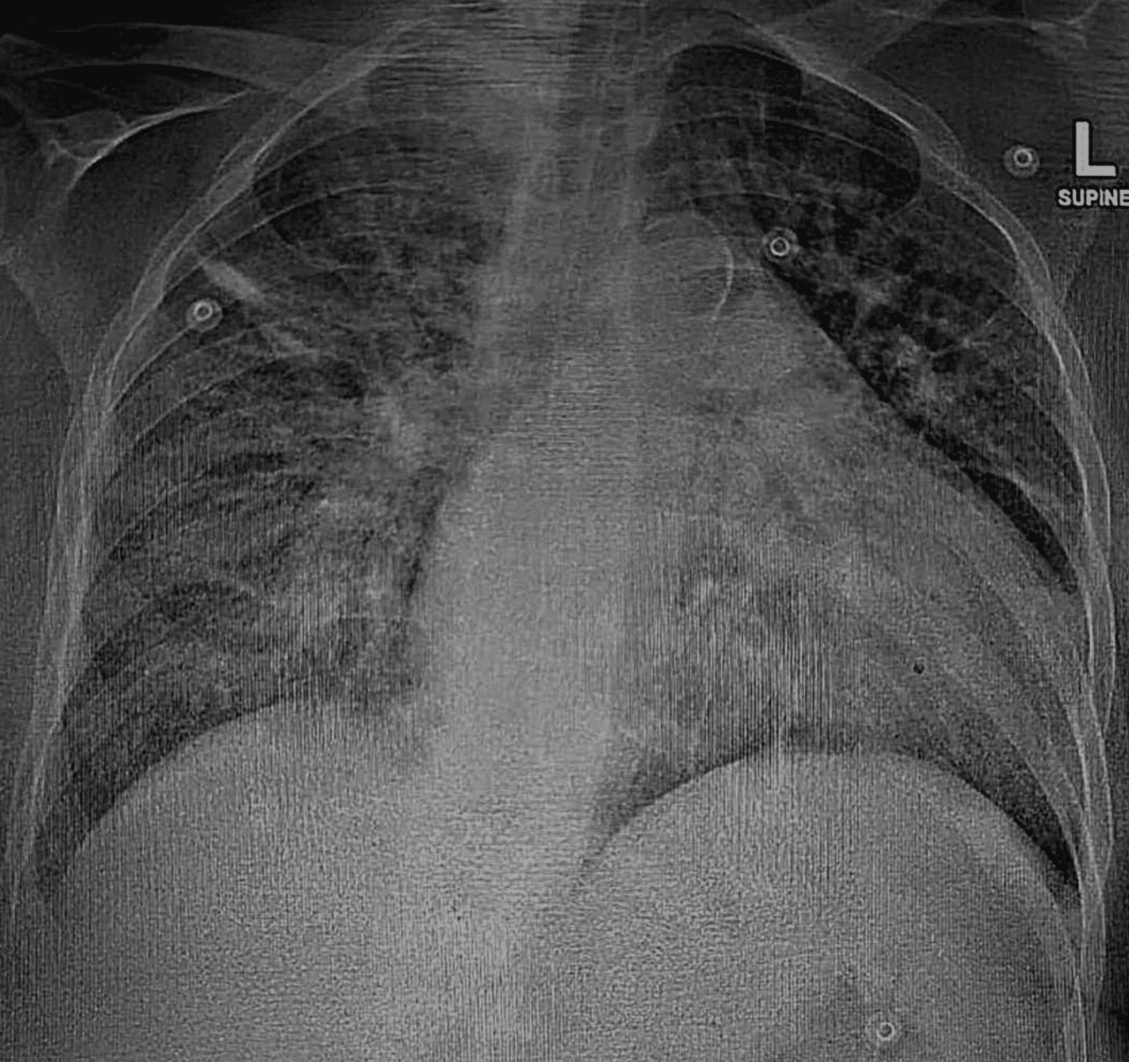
Chest X-ray Confirming nasogastric tube position with no acute pulmonary findings.
